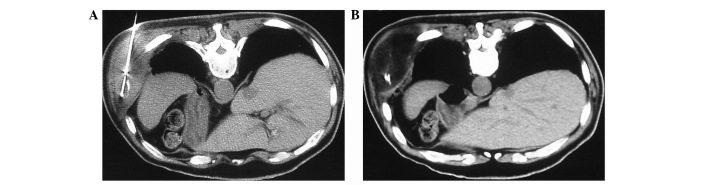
Lung squamous carcinoma with rib metastasis. (A) Cryoprobes inserted into metastatic lesions under CT scan; (B) monitoring the area of ablation by CT scan. CT, computed tomography.
